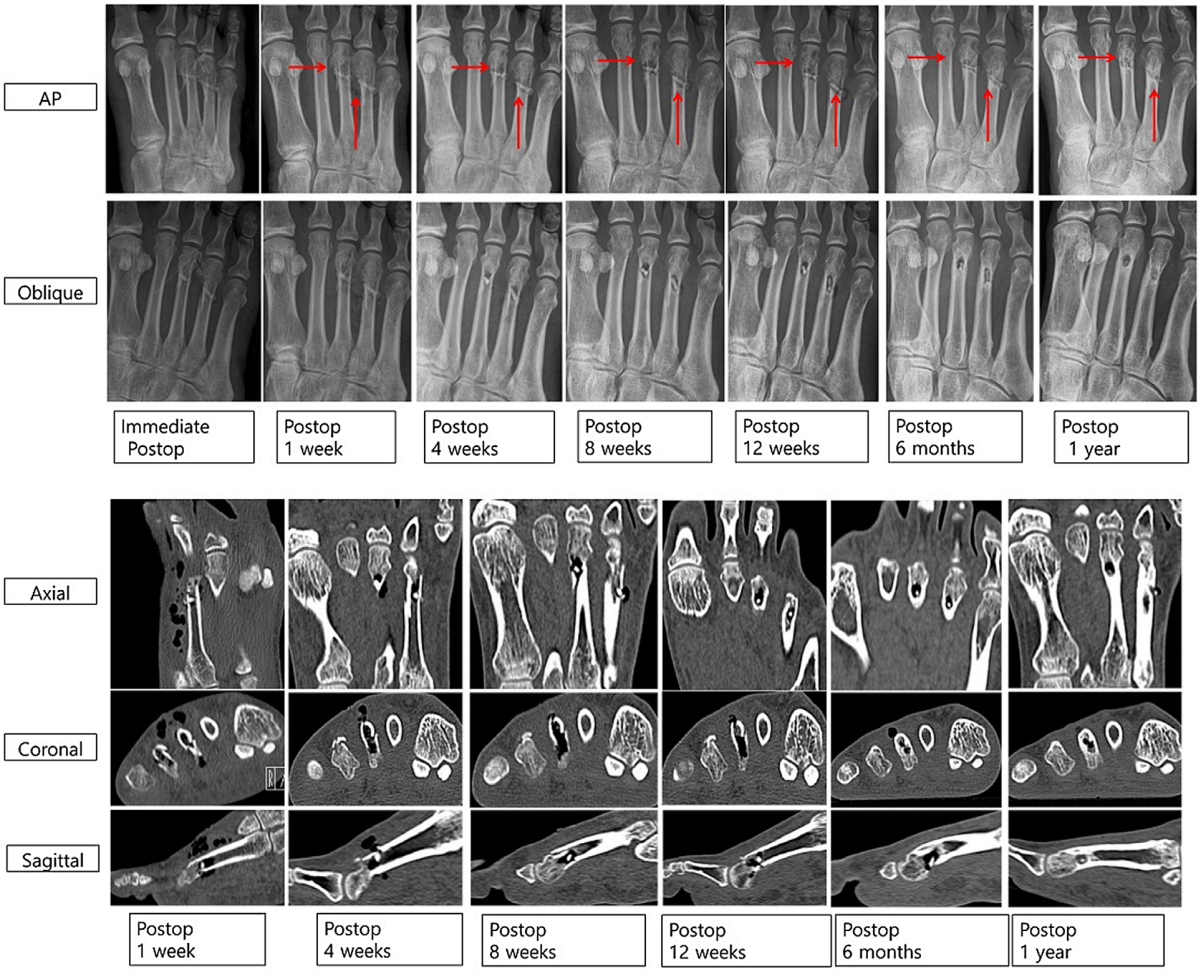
Foot AP and oblique X-ray images of gas formation by magnesium screw (red arrows), and axial, coronal, and sagittal CT scan images of air bubbles surrounding magnesium screw inserted into metatarsal fracture for 12 months.
